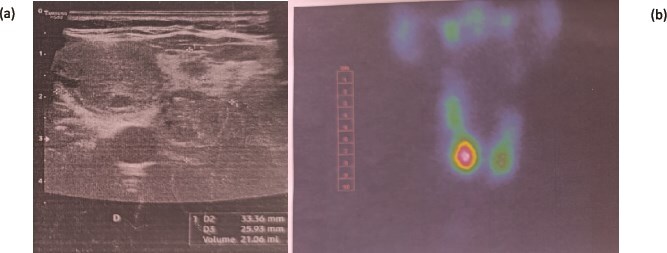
Ultrasound (a) and Tc-99 m thyroid scintigraphy (b) of our patient.
